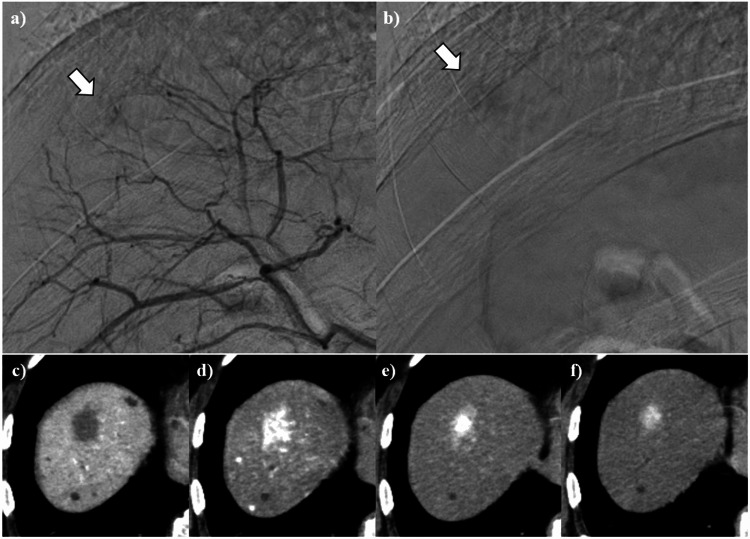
Hepatic arterial angiography. Hepatic arterial angiography of the hepatic nodule. The lesion showed persistent enhancement from the early to late phase (arrows) (a,b). CT angiography showed the lesion non-enhancement in the portal phase (c), while the lesion showed persistent enhancement during all hepatic arterial phases: (d) the early, (e) the middle, and (f) the delayed components.
